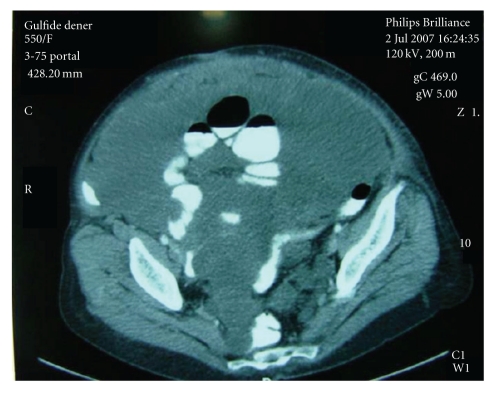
Computed tomographic features of the case. A very large incisional hernia is seen. Bowel loops float within mucinous ascites.
